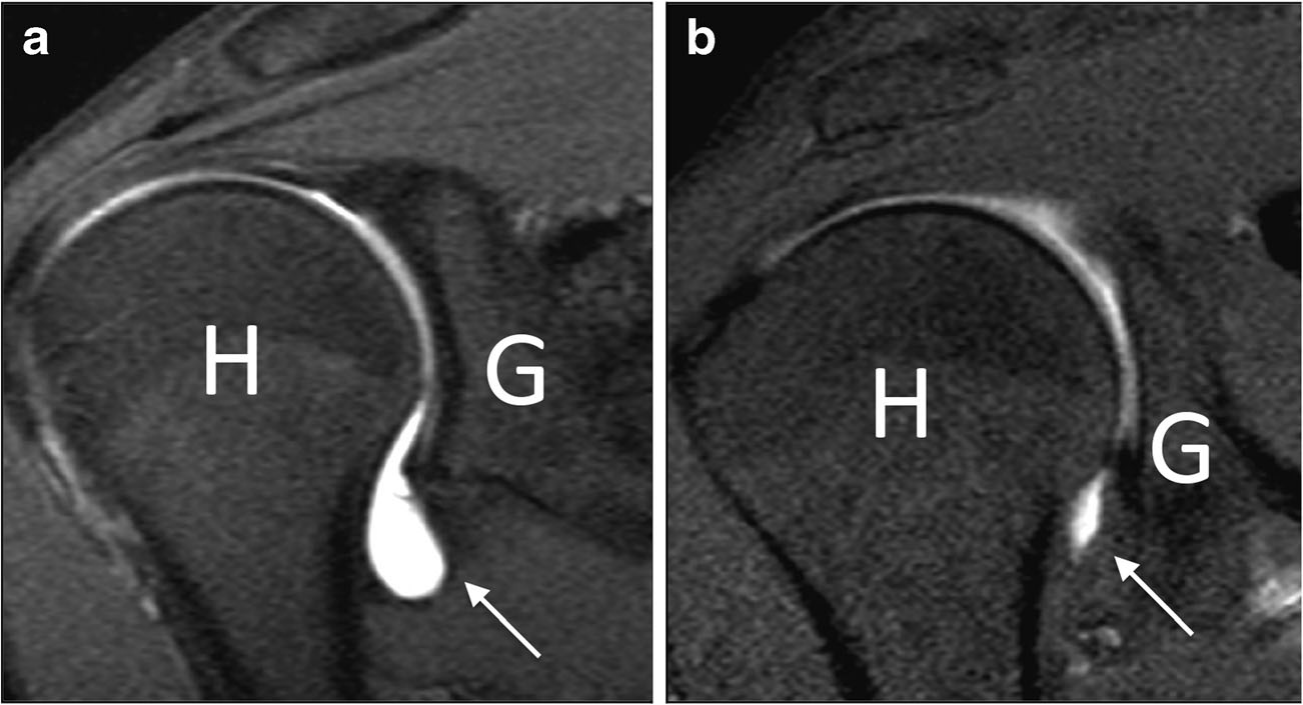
MR arthrography, coronal oblique T1-weighted fat-saturated image. (a) In a healthy subject, the axillary pouch is normally distensible (*arrow*). *H* humerus, *G* glenoid. (b). In a patient with adhesive capsulitis, the axillary pouch is contracted and poorly distended (*arrow*)
